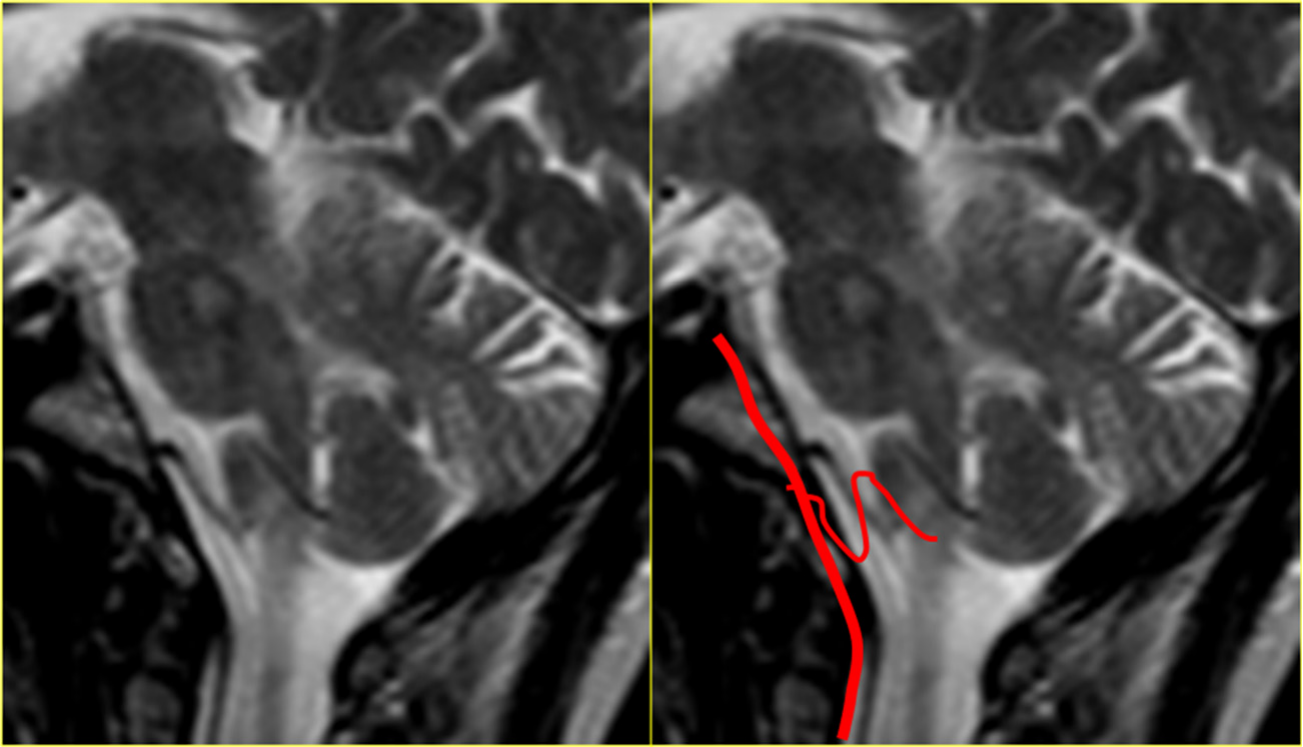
Magnetic resonance imaging scans: T2-weighted image, sagittal plane. Scan shows the vertebrobasilar system. The posterior inferior cerebellar artery (PICA) arises from the vertebral artery. The upward buckle almost reaches the caudal pons.
